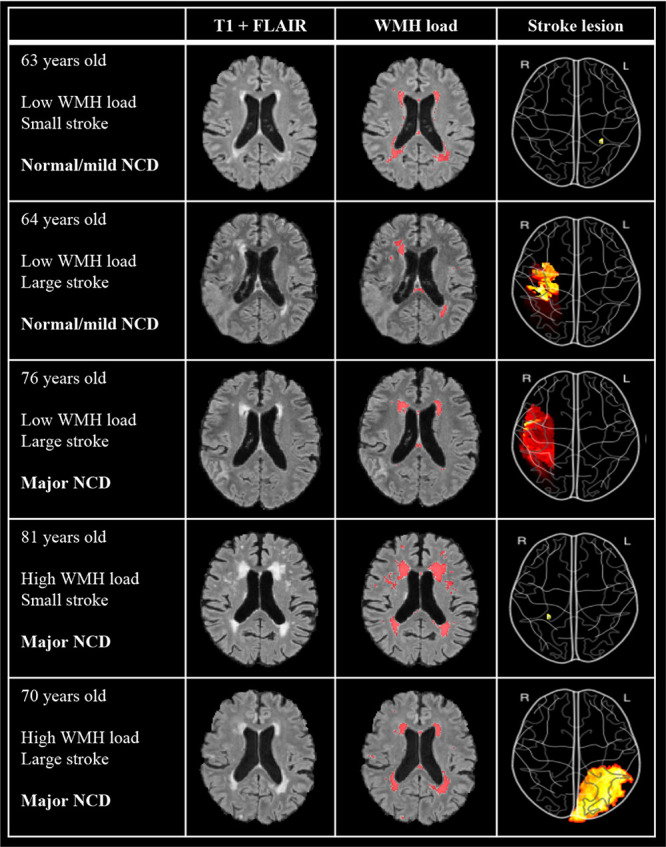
Examples of imaging findings of five participants with differing levels of WMH, stroke volumes, and NCD outcome. T1 + FLAIR image shows “untouched” co-registered image and WMH load shows the same co-registered image with annotated WMH from manually edited output from FSL BIANCA. Stroke lesion color map depicts volume projection, such that red indicates a larger stroke than yellow. Image is flipped so as to correspond to the hemisphere of MRI images. WMH, white matter hyperintensities; NCD, neurocognitive disorder.
